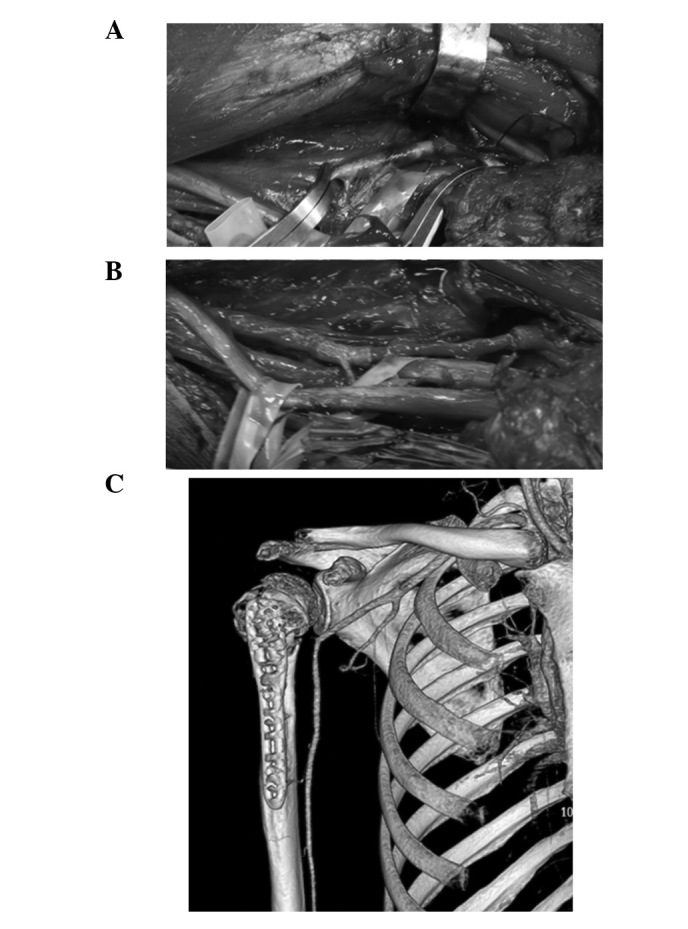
Reconstruction of the injured axillary artery. (A) Intraoperative photograph of the thrombosed axillary artery. (B) Intraoperative photograph showing an interposition graft with a saphenous vein. (C) CTA 3 months after surgery showing repaired artery. CTA, computerized tomography angiography.
